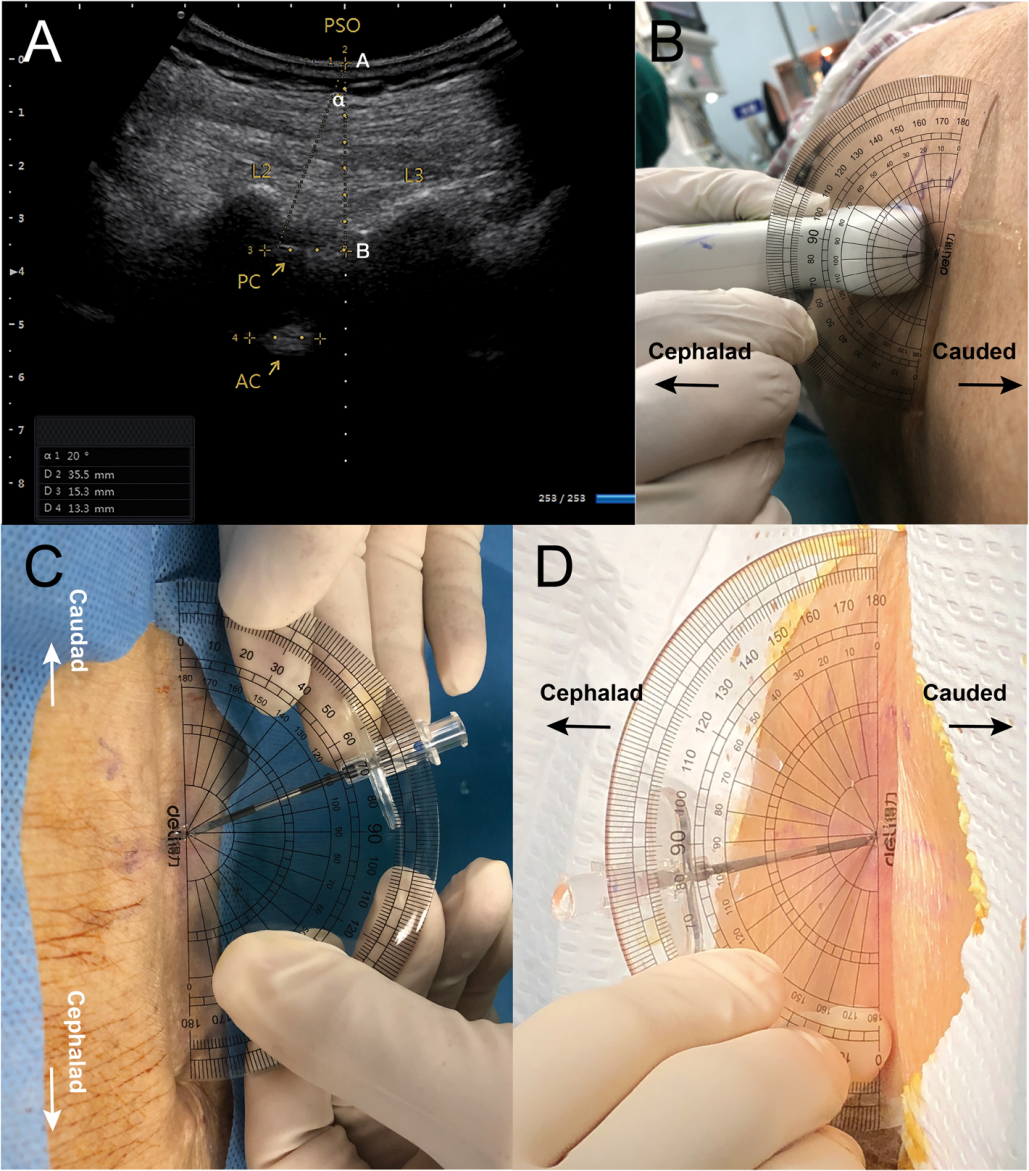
Measurement and application of the needle angle. a An ultrasound image from the paramedian sagittal oblique (PSO) view, which shows the L2-L3 interlaminar space, posterior complex (PC), and anterior complex (AC). ∠α is the measured maximum cephalad angle of the needle. A to B is the distance from the skin to the posterior complex. b Measuring the medial angle with a 180° protractor during the paramedian approach, which is the tilting angle of the probe to the median plane. c An aseptic 180° protractor was used to assist the cephalad needle insertion angle. d After a successful puncture, the actual medial angle was measured with an aseptic 180° protractor
